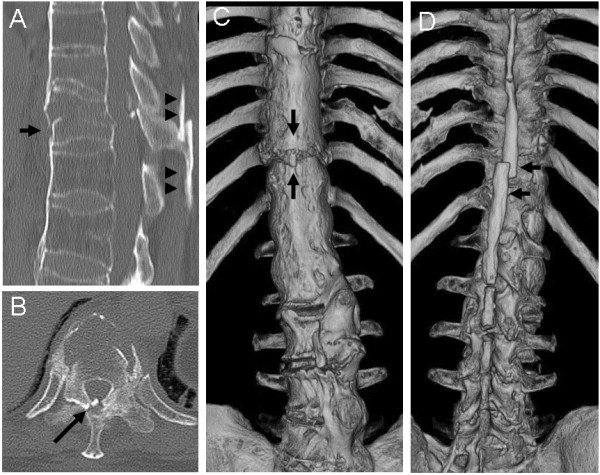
Computed tomography taken when a patient with Parkinson’s disease was first referred to our hospital eight days after onset of back pain. The sagittal image (A) shows a fracture of the T11 vertebral body (arrow) and displacement of ankylosed spinous processes (arrowheads). The axial image (B) shows ossification of the ligamentum flavum at the T10-T11 level (arrow). Three-dimensional images (C, D) show a severely ankylosed spine at the thoracolumbar levels, and the fracture site is clearly seen (arrows).
